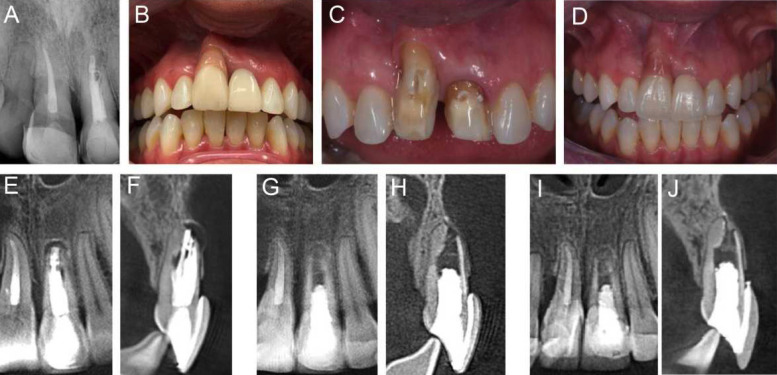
A) Initial periapical radiograph showing a sizeable inadequately-filled canal space associated with a discrete periapical radiolucent lesion on tooth # 21. B) Preoperative clinical aspect. C,D) Coronal reconstruction of teeth #8 and #9 with esthetic restoration. E, F) Preoperative cone-beam computed tomography (CBCT) images. Teeth #9 showed conventional root canal therapy and showed a large inadequately filled canal space associated with a periapical radiolucent lesion. Postoperative CBCT of tooth #9 eight months (G,H) and 34 months (I,J) after RET showing complete repair of the apical periodontitis lesion.
